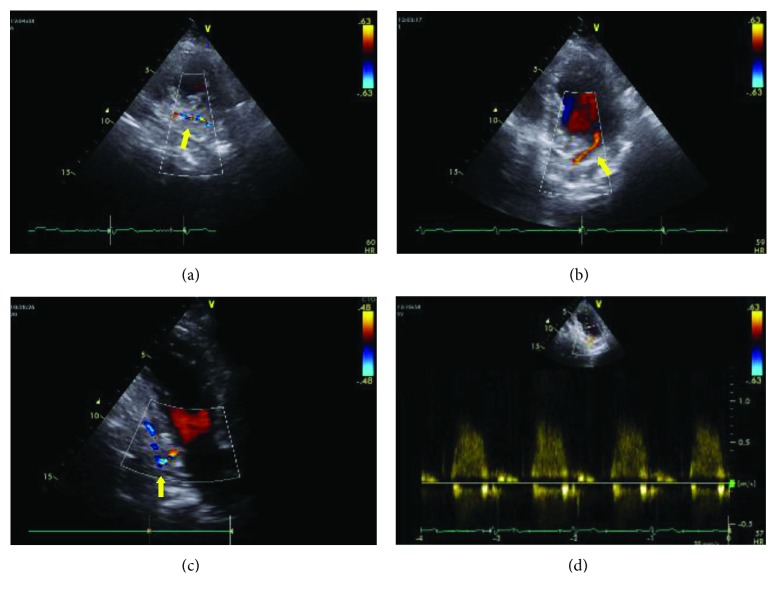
Midventricular (a) and basal (b) short axis view and 2-chamber (c) with color Doppler, showing fistula's course in the left ventricle inferoposterior wall (arrows). (d) PW-Doppler recording fistula flow that is predominantly diastolic.
